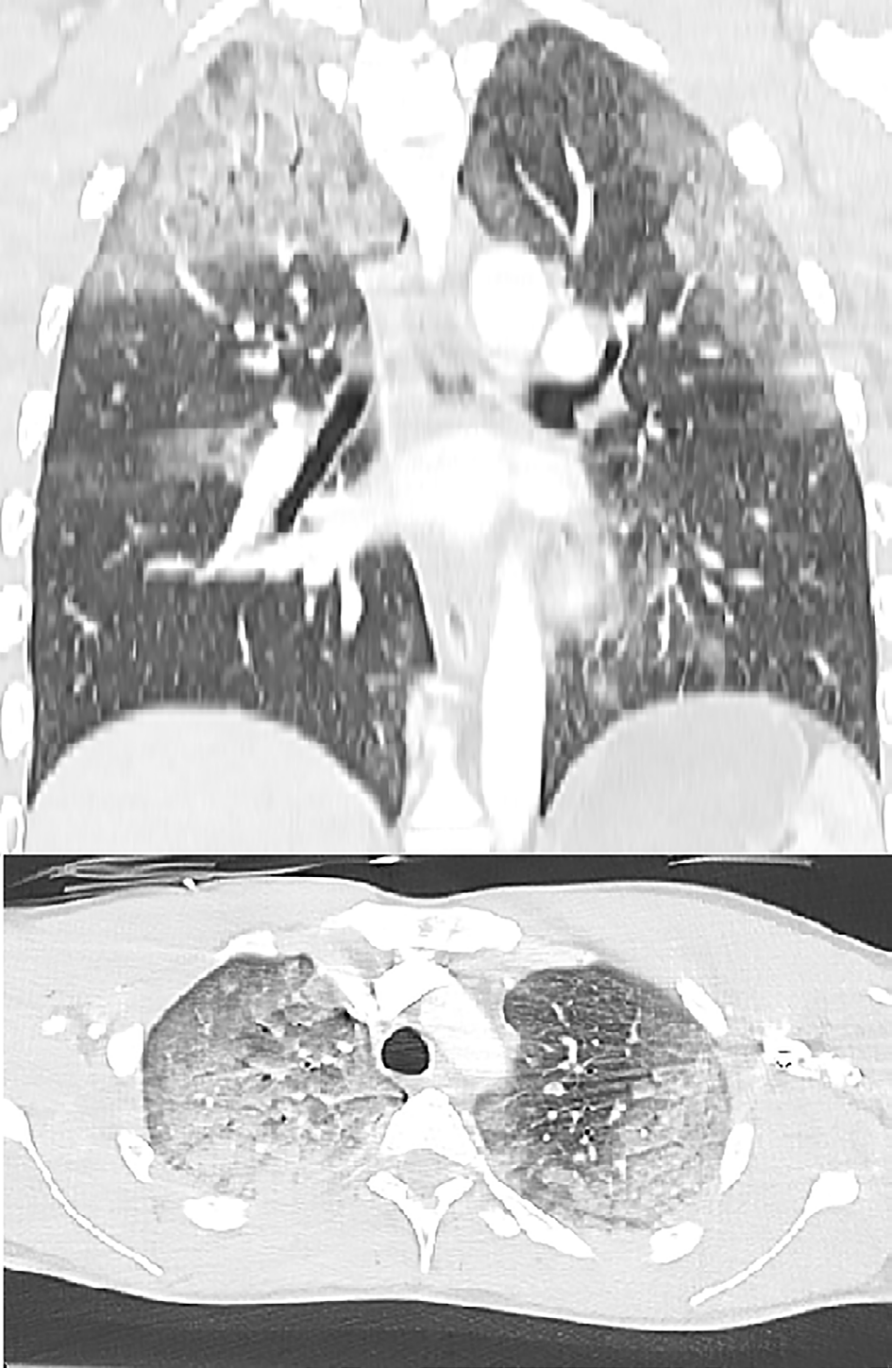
Chest CT: ground glass and consolidation, most pronounced in the upper lobes.
